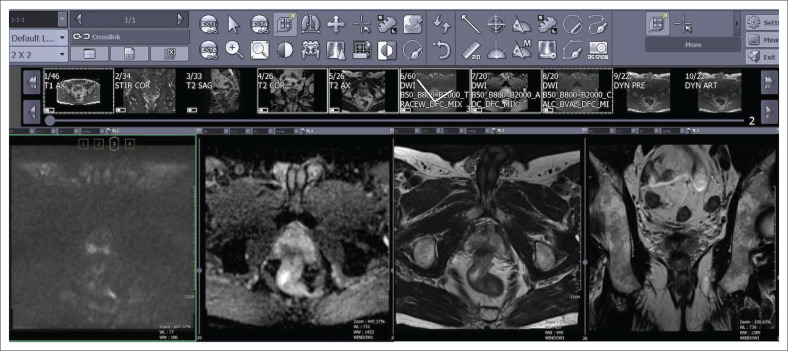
Workstation layout of four sequences used for multiparametric prostate magnetic resonance imaging evaluation of the prostate including (left to right) high *b*-value diffusion-weighted imaging, Apparent Diffusion Coefficient (ADC) map, axial and coronal T2-weighted imaging.
